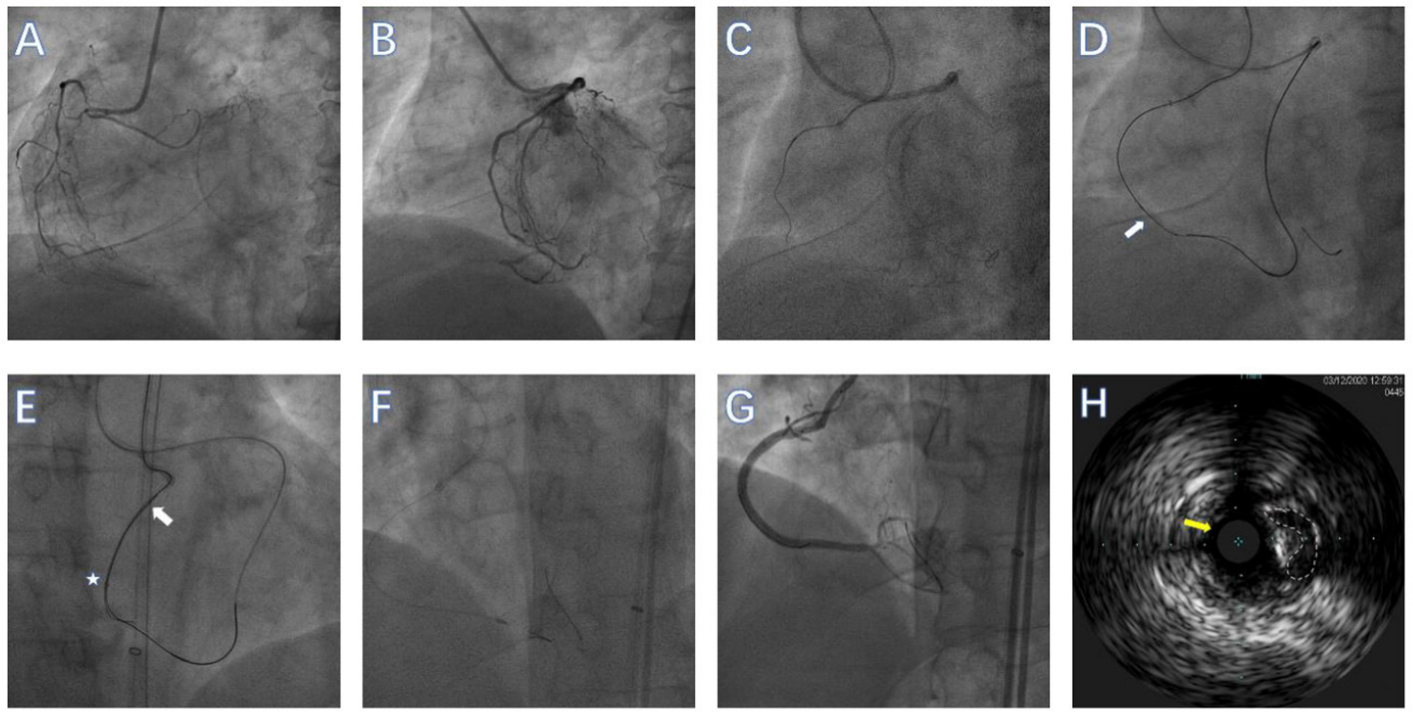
Performing Guidezilla^TM^ reverse controlled antegrade and retrograde subintimal tracking (CART) during rotational atherectomy (RA) in a right coronary artery (RCA) CTO lesion. (A) RCA in left anterior oblique view showing an ambiguous proximal cap without obvious calcification. (B) RCA in left anterior oblique view showing the distal cap of the RCA. (C) Antegrade wire in the subadventitial space of mid-RCA. (D) The CTO lesion was crossed over using the Guidezilla^TM^ reverse CART technique (the white arrow represents the dilated balloon). (E) Retrograde wire (white arrow) was advanced into the Guidezilla^TM^ (white star) of the RCA and externalized. (F) Rotational atherectomy was then performed using a 1.25-mm burr. (G) Angiography after successful CTO-PCI. (H) Intravascular ultrasound confirmed that the guidewire (yellow arrow) was in the subadventitial space (The white dotted line represents the true lumen of the vessel).
